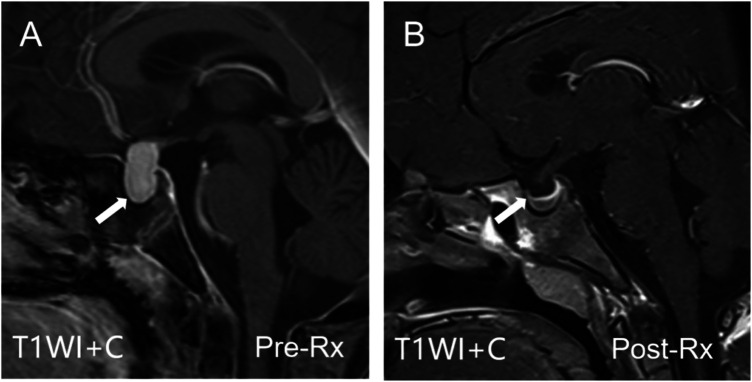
Pituitary MRI with contrast enhancement. (A) Sagittal contrast-enhanced T1-weighted image showing marked symmetrical pituitary enlargement (white arrow) with upward convexity and a characteristic “waist sign,” indicative of pituitary hyperplasia. (B) Follow-up after 4 weeks of levothyroxine therapy demonstrating significant reduction in pituitary size and near-complete restoration of normal morphology.
